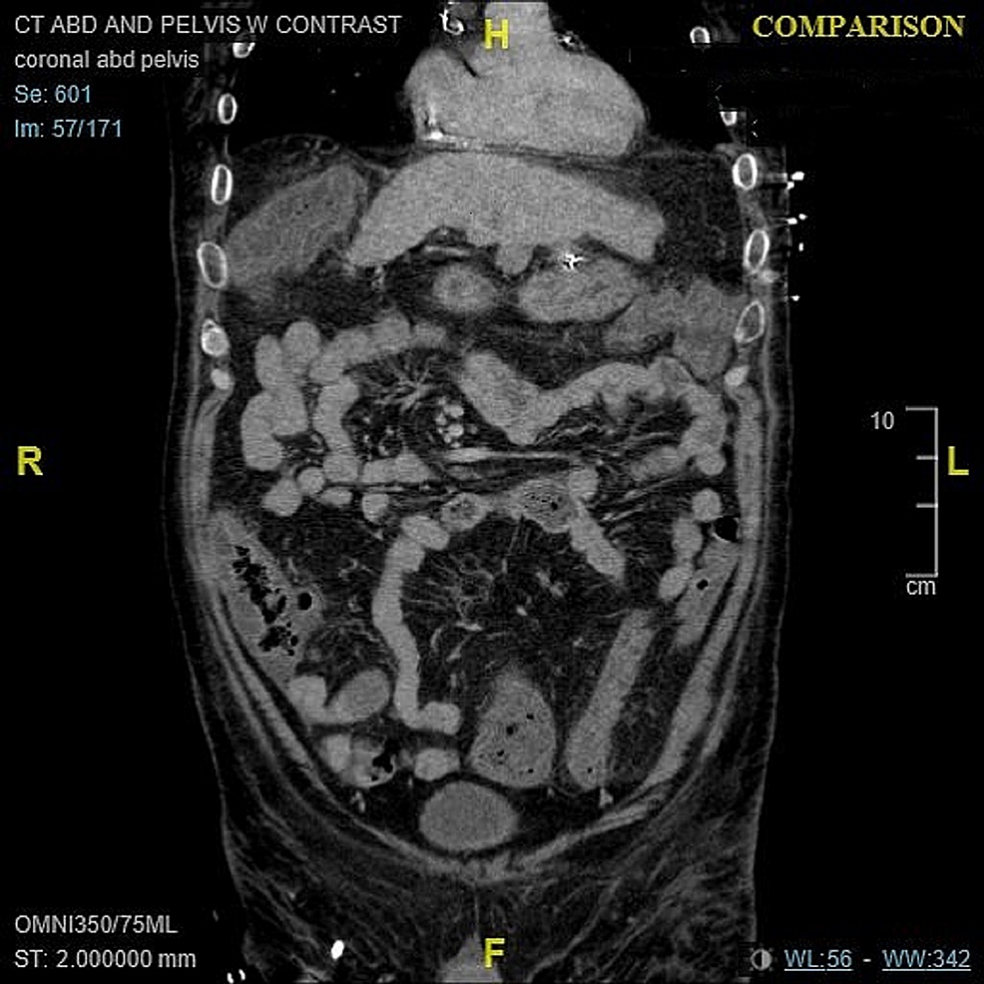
CT of the abdomen/pelvis on admission. Coronal view: On admission, marked diffuse colonic wall thickening with adjacent fat stranding, suggestive of colitis without dilated bowel (maximum right colon dilation of 4 cm), and nodular contour of the liver, suggestive of hepatic cirrhosis. CT: computed tomography
